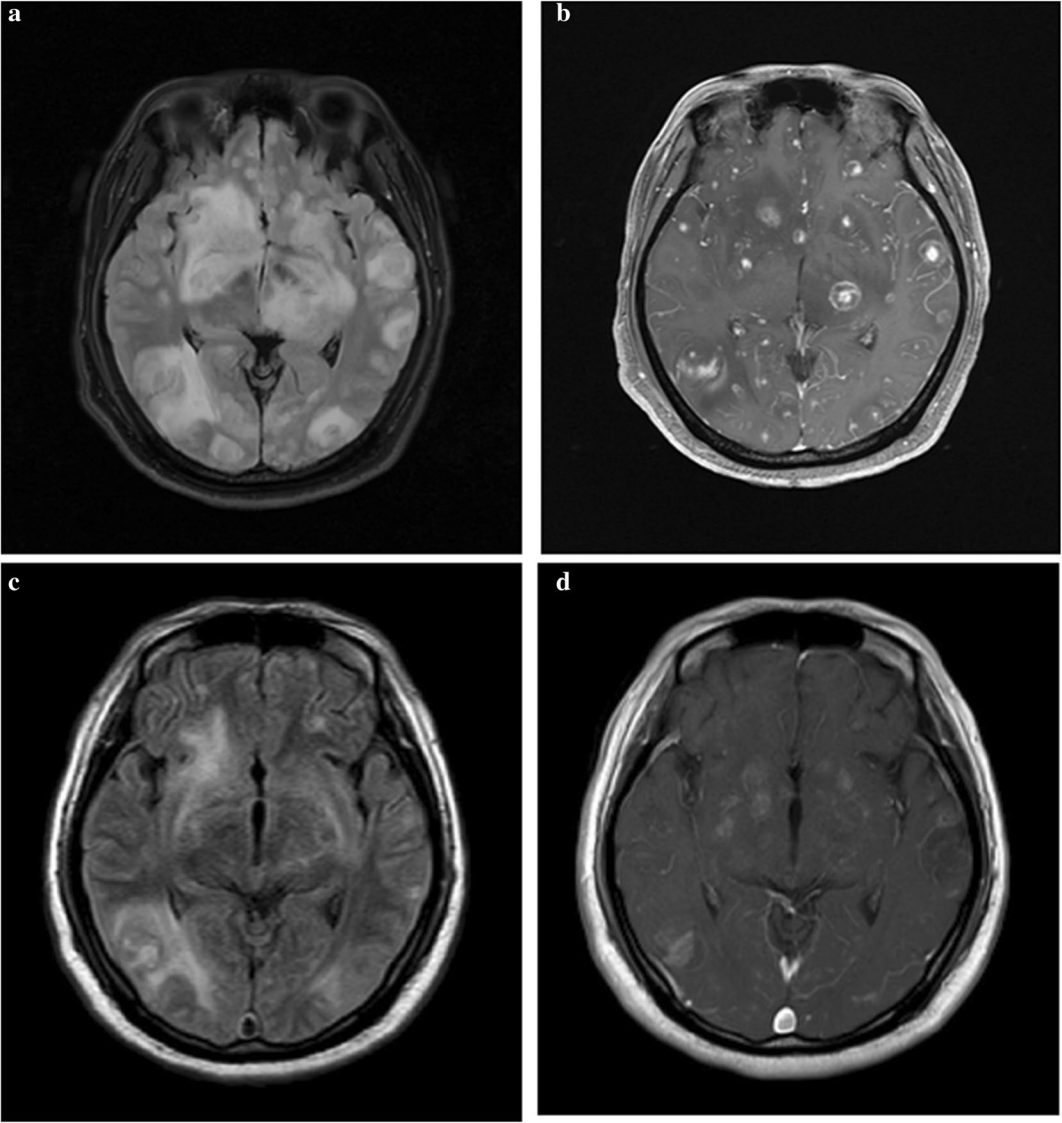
Brain magnetic resonance imaging before and after anti-*Toxoplasma gondii* therapy. At admission, brain magnetic resonance imaging (MRI) showed multiple brain abnormalities (a T2-FLARE imaging) with enhancement (b contrast-enhanced MRI). After treated as toxoplasmosis encephalitis for 11 days, a following-up brain MRI showed significant improvement of brain lesions(c T2-FLARE imaging; d contrast-enhanced MRI)
